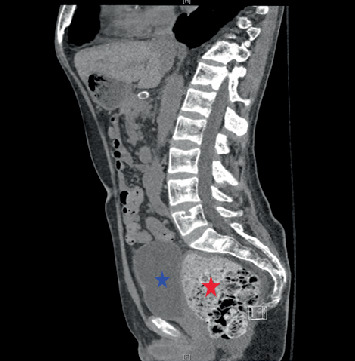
CT scan of the abdomen and pelvis without contrast showing a large fecal impaction (red star) and, in this view, shown to be causing compression of the urinary bladder (blue star).
